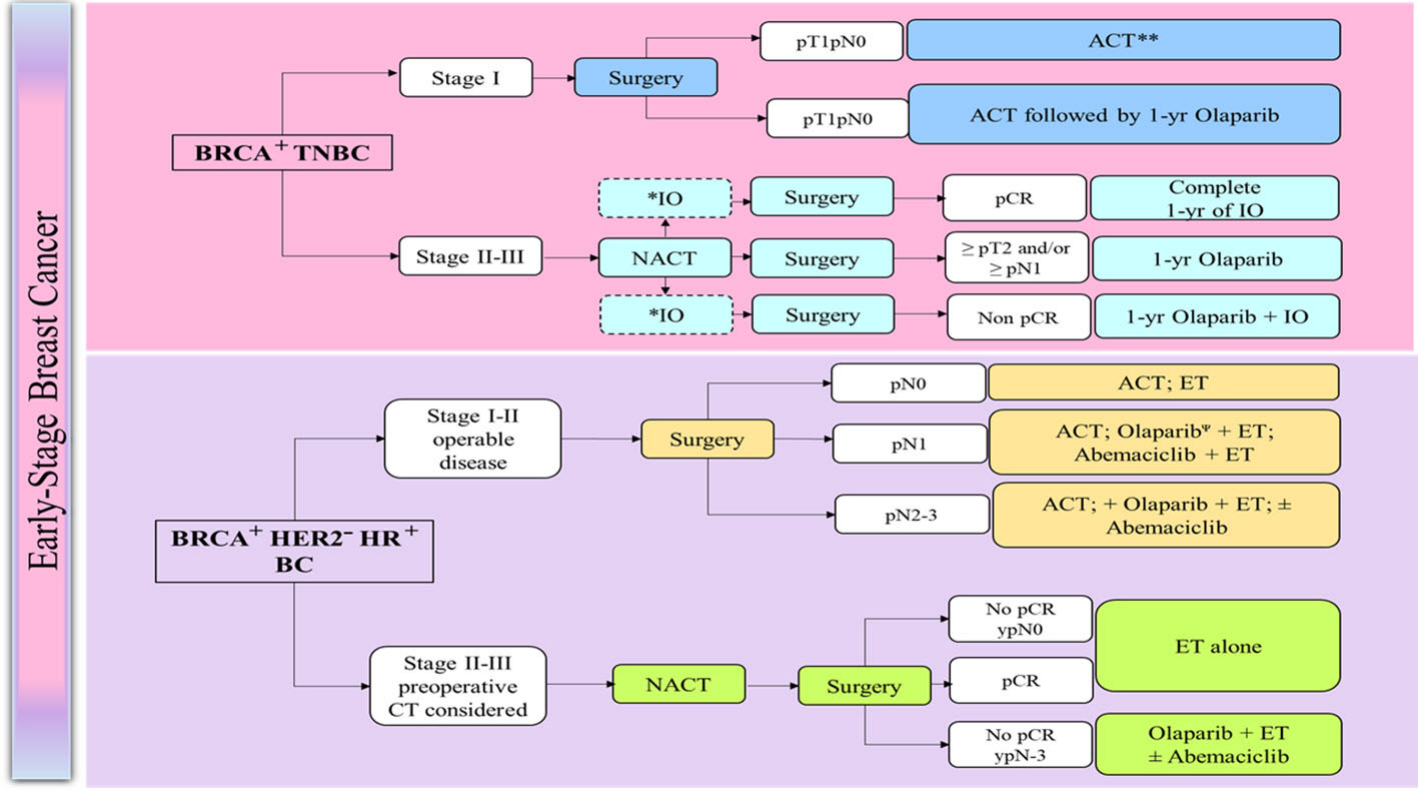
Management of early breast cancer in germline *BRCA1/2* mutation carriers (129). ACT, Adjuvant chemotherapy; BRCA, BReast CAncer gene; CT, chemotherapy; CPS + EG, Combined Positive Score; clinical-pathologic staging system incorporating estrogen receptor-negative disease and nuclear grade 3 tumor pathology; ER, estrogen receptor; ET, endocrine therapy; HR, Harmone receptor; HER2, human epidermal growth factor receptor 2; NACT, Neoadjuvant chemotherapy; N0, node-negative; LN, Lymph node; pCR, Pathological complete response; TNBC, triple-negative breast cancer. *: PDL1 positive; : case-by case for pT1a, Ψ: Preferred after chemotherapy, case-by-case for pT1a.
